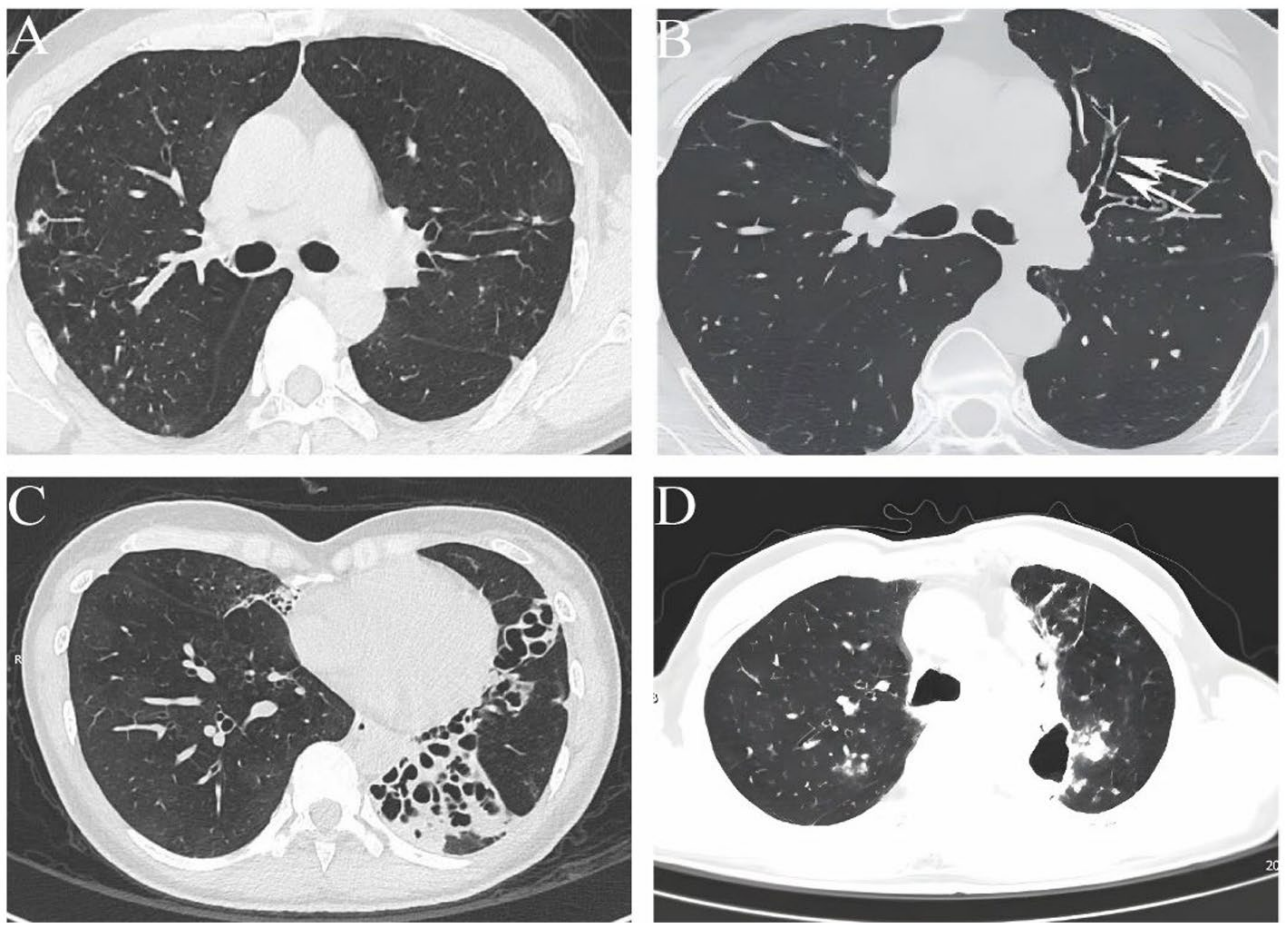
Abnormal pulmonary computed tomography images in patients with bronchiectasis complicated by *M. avium* complex infection. (A) Nodular lesions and bronchiectasis. (B) Cylindrical bronchiectasis. (C) Cystic bronchiectasis. (D) Thin-walled cavity.
